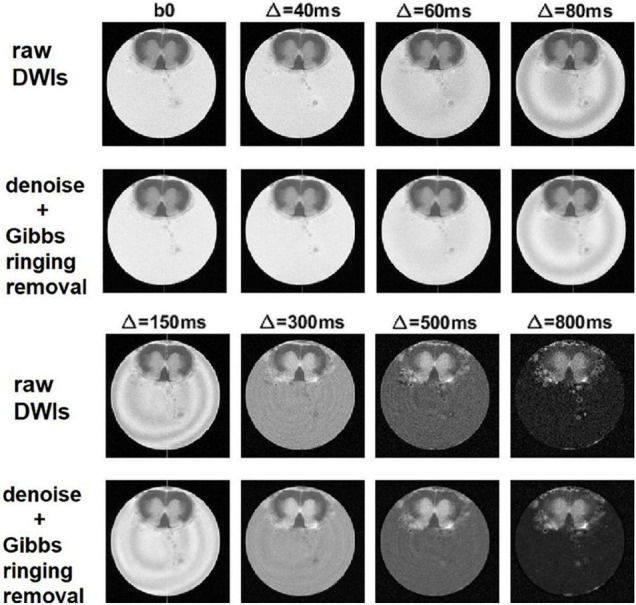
Denoising and removal of Gibbs ringing artifact in DWI used to obtain α-imaging. Comparison between the raw DWIs and the DWIs after denoising and the removal of Gibbs ringing artifact, for the lumbar slice of the spinal cord, considering the DWIs acquired along z-direction and using increasing diffusion time, Δ.
